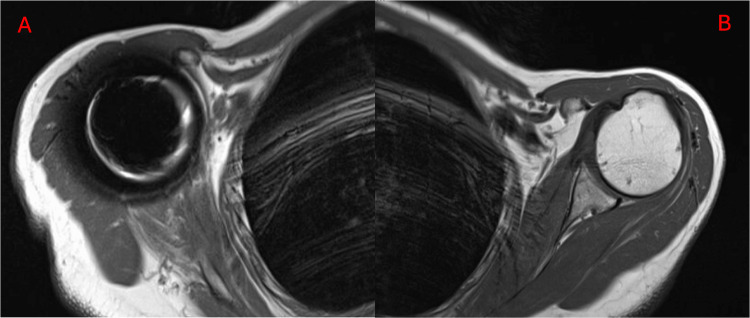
MRI at 4-year Follow-up Displaying Mild Fatty Infiltration and Atrophy of the Right Deltoid Muscle (A) Compared to Healthy Left Shoulder (B)
